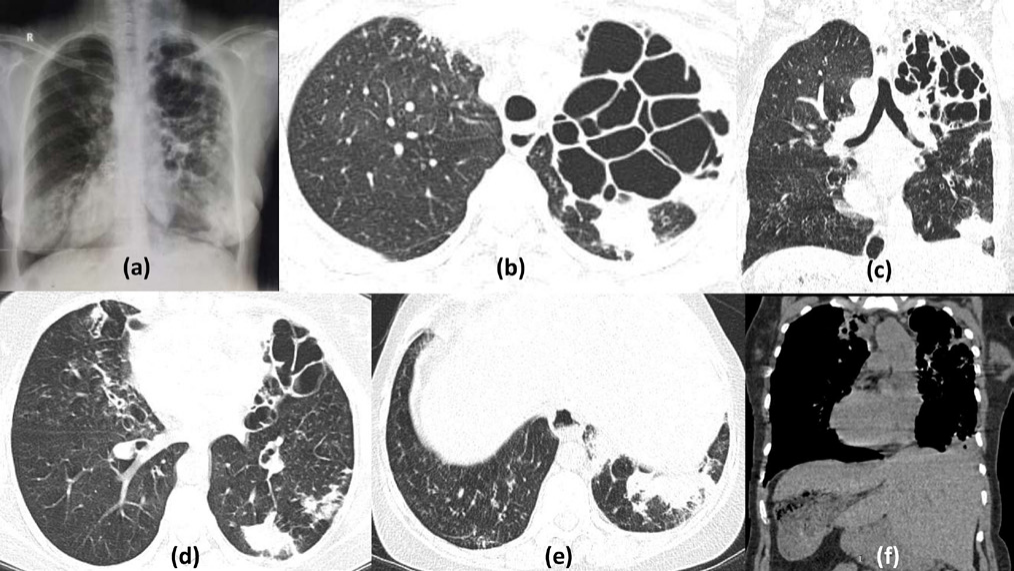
Cystic bronchiectasis with COVID19 infection in Kartagener Syndrome: 26-year-old female with chronic sinusitis and low-grade fever with cough, tested positive for COVID19 infection. Frontal radiograph (a) shows bilateral asymmetrical bronchiectasis with patchy peripheral air space opacities in the left lower zone. Note is made of situs inversus. Chest HRCT done on Day 20 of illness, axial sections (b, d and e) show patchy peripheral consolidations with extensive cystic bronchiectasis involving the entire left upper lobe and right middle lobe. Coronal lung (c) and mediastinal window (f) sections reveal bilateral hyparterial bronchus, dextrocardia, left-sided liver with right-sided stomach suggestive of complete situs inversus.
